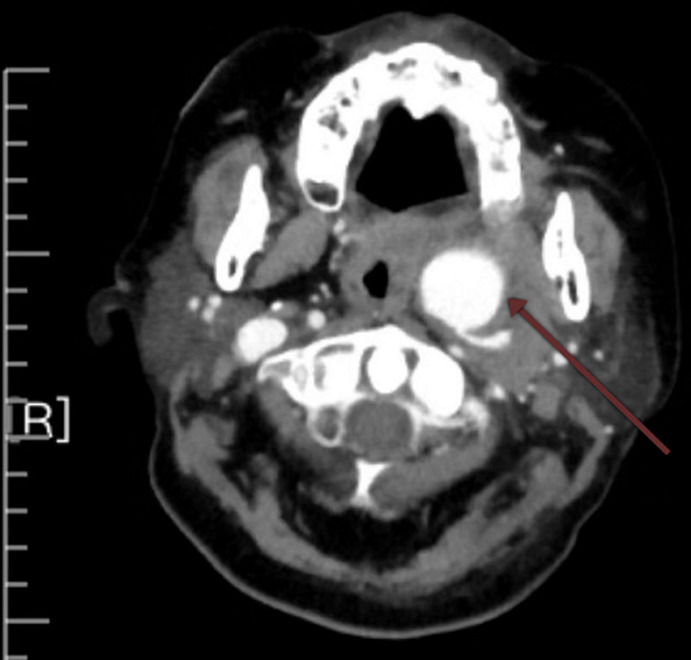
neck CT showing a high-density shadow measuring 2.9 × 3.7 cm at the area of the ICA, parapharyngeal space and pharyngeal space
